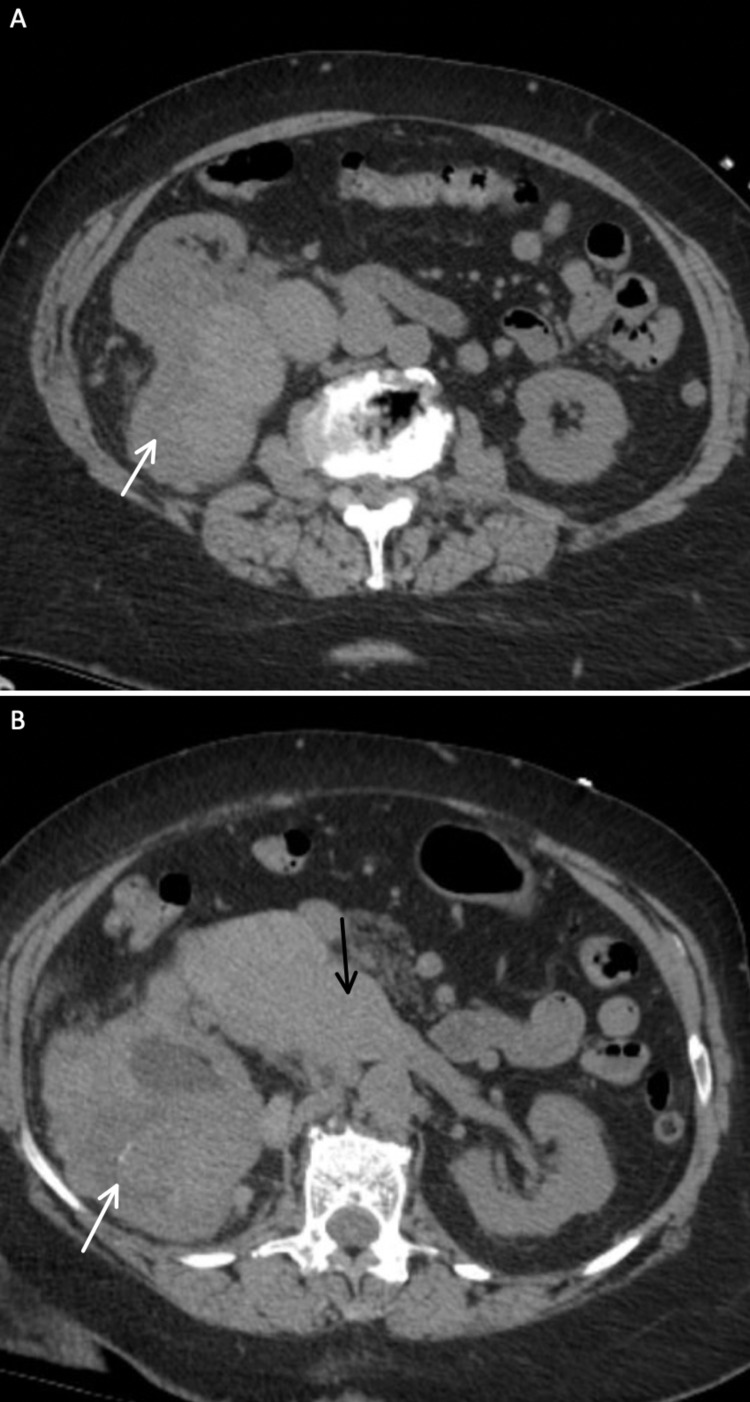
Axial View of the Right-sided Renal Mass Axial computed tomography (CT) images (A, B) showing a large multilobulated 12.8 cm x 9.3 cm solid and cystic right-sided renal mass and enlargement of the renal vein suggestive of tumor thrombus. The white arrows point towards the renal mass. The black arrow points towards the tumor thrombus extension into the renal vein and inferior vena cava.
